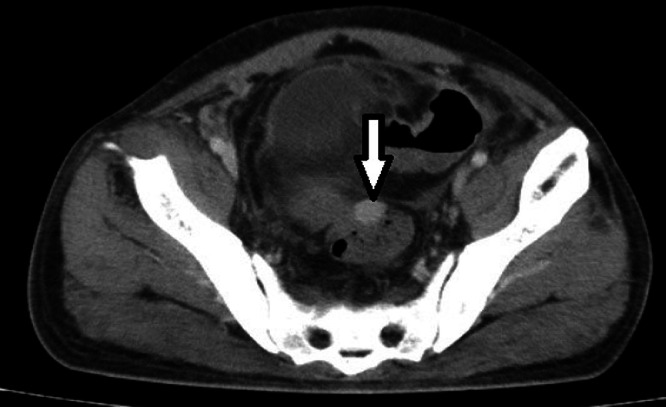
Axial contrast enhanced CT image showing splenic implant in pelvis abutting sigmoid colon.
